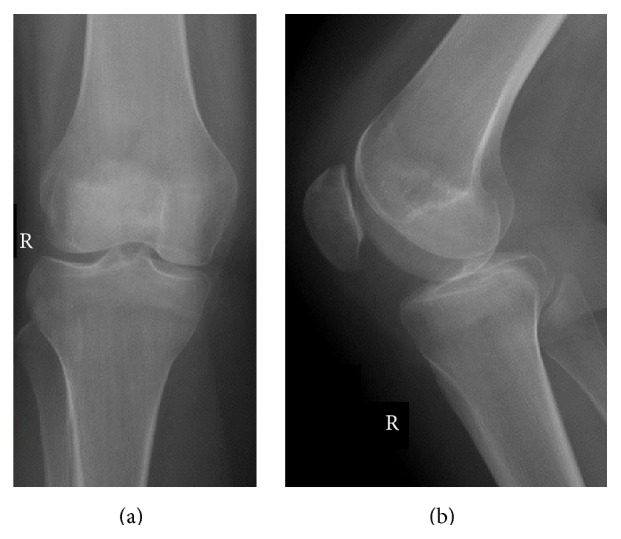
Anteroposterior (a) and lateral (b) follow-up X-rays after the second operation.
